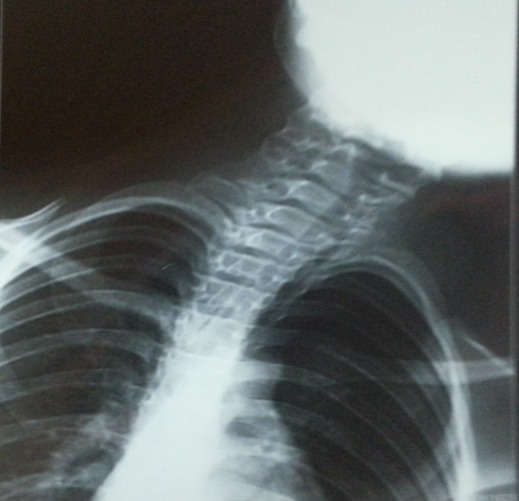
Scoliose a la radiographie thoracique
